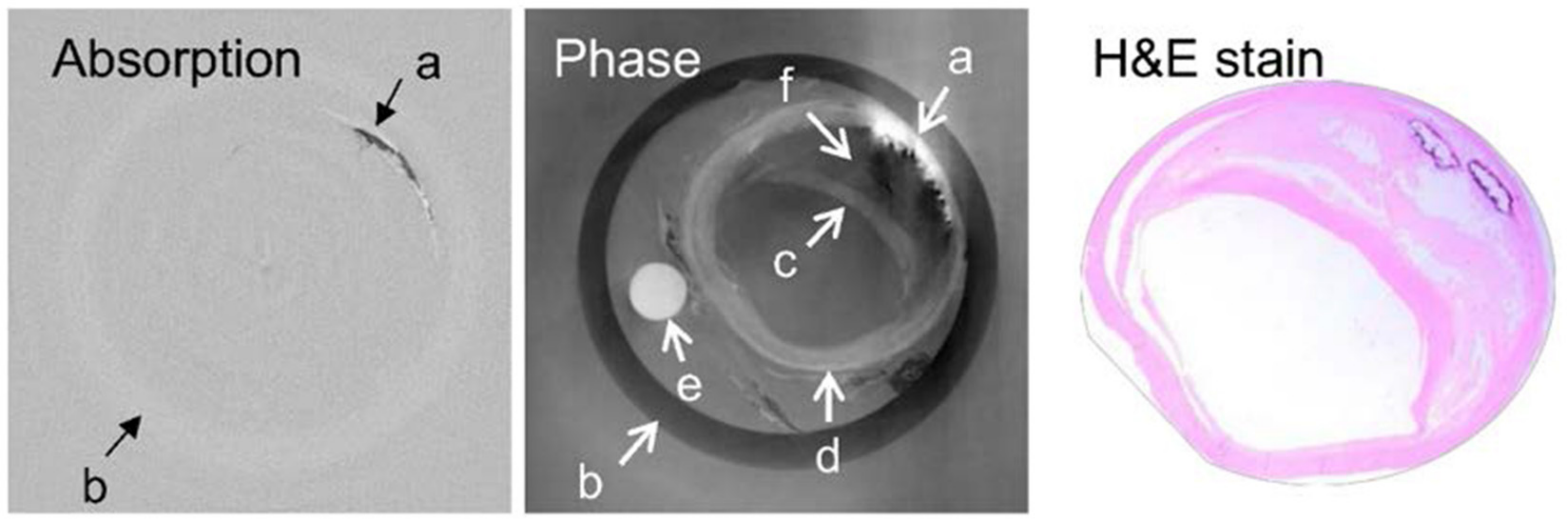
Improved soft-tissue contrast afforded by X-ray phase images: An iliac artery with extensive atherosclerotic disease demonstrating different plaque components in the phase but not attenuation image a: calcification; b: plastic container; c: fibrous cap; d: tri-laminar arterial wall; e: a plastic rod as fiducial marker; f: soft plaque or atheroma.
